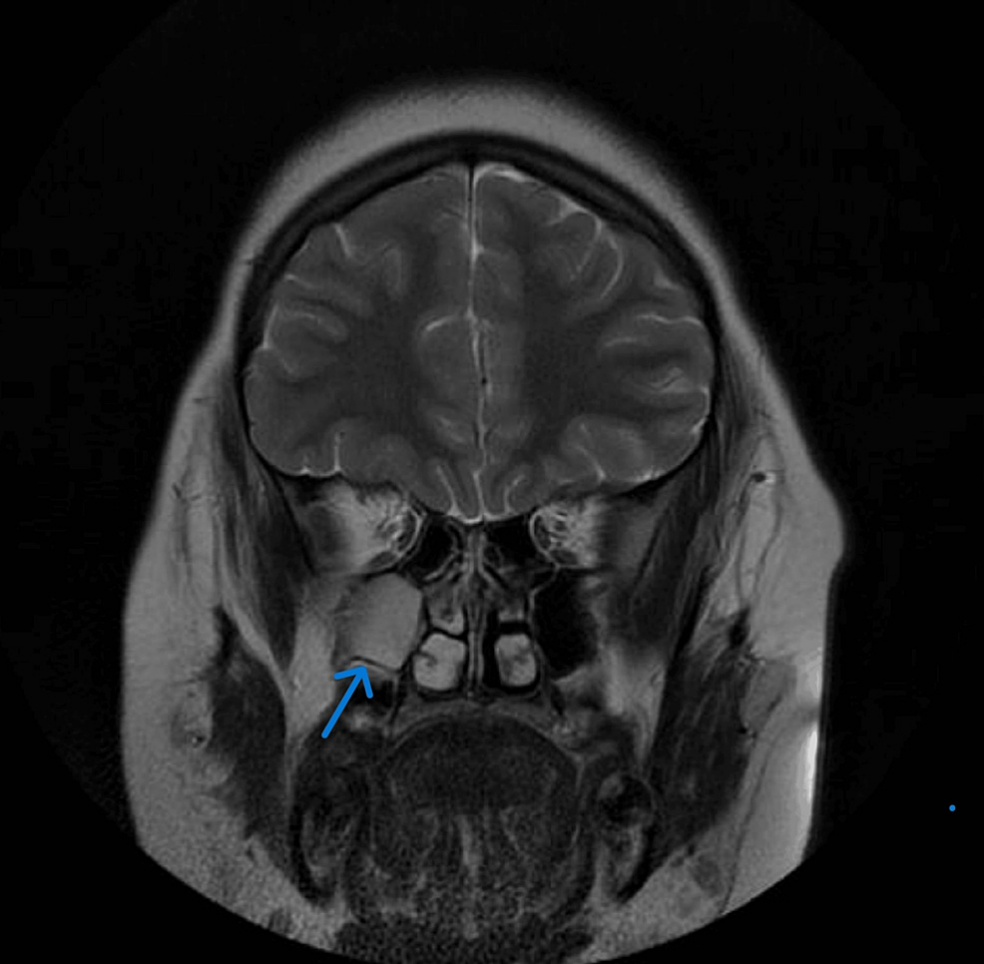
MRI brain scan with T2-weighted image, a sagittal section with a 3D view showing the right maxillary retention cyst
